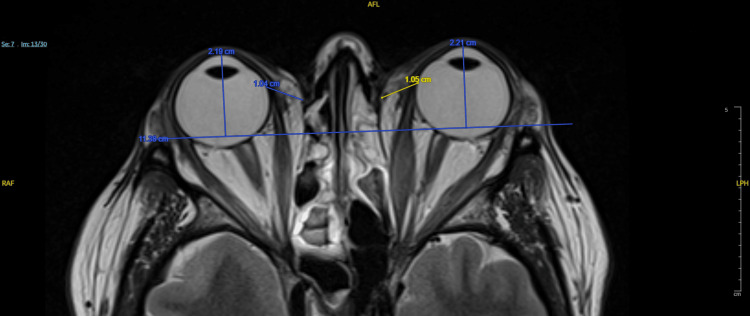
Measurement of exophthalmos (mm) and orbital fat thickness (mm) on axial T2WI T2WI: T2-weighted image
